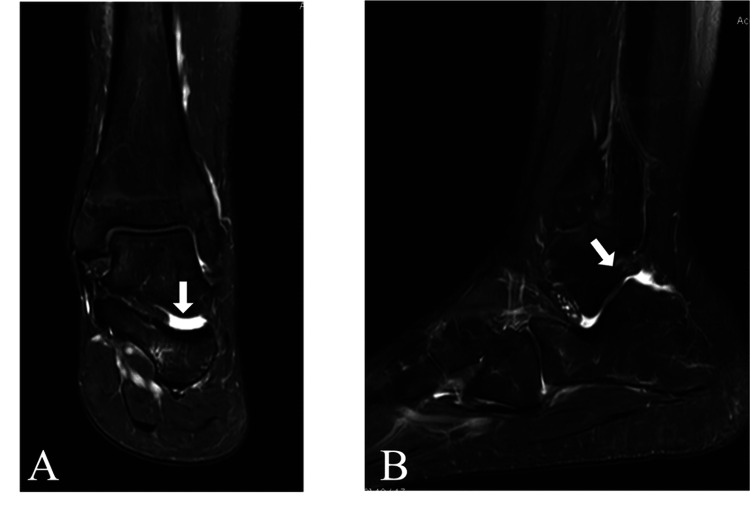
Magnetic resonance imaging (MRI) findings of the subtalar joint (A) Coronal view; (B) Sagittal view. Arrows indicate hyperintense signals suggestive of joint effusion within the subtalar joint.
